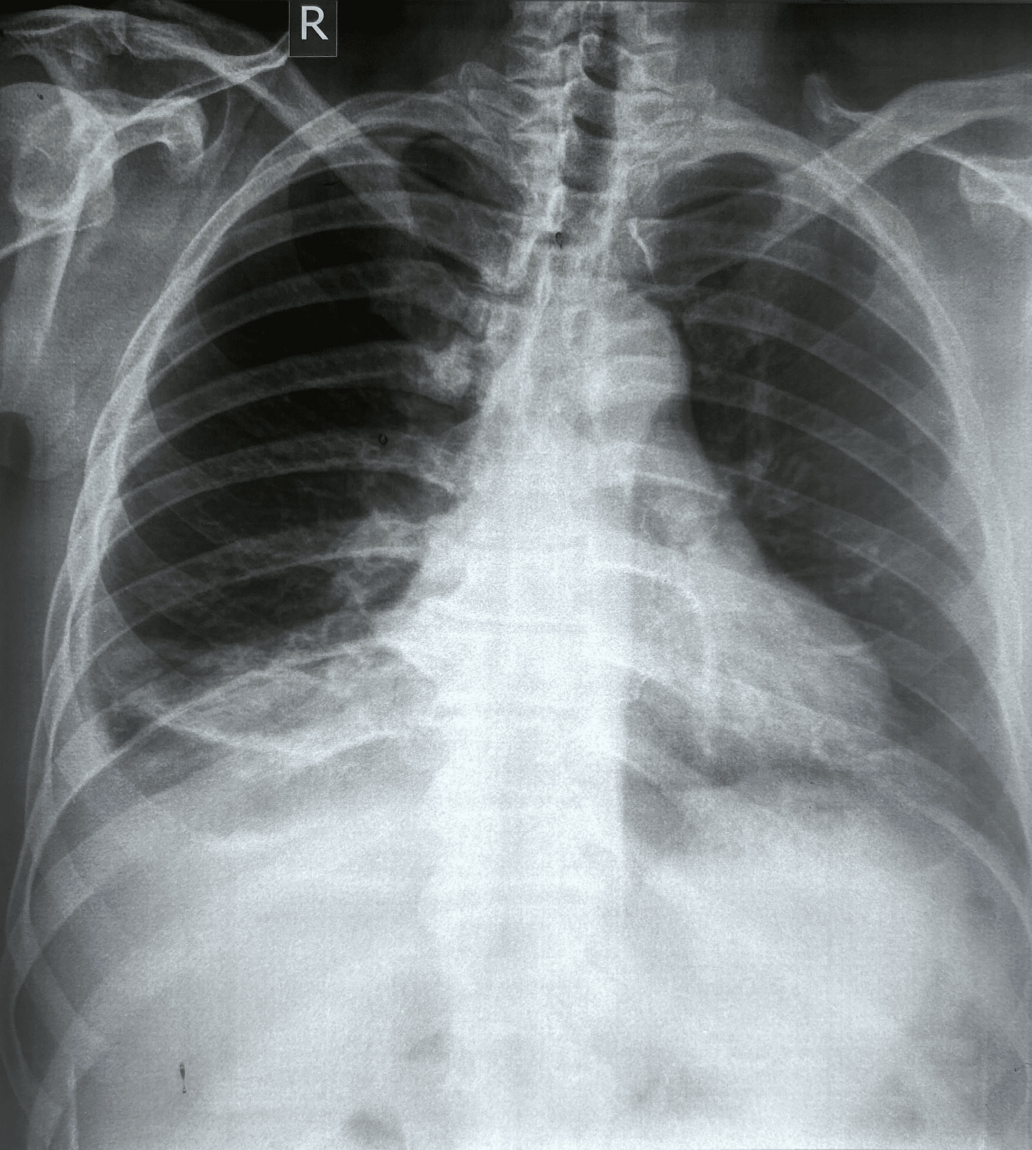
Follow-up Chest X-ray shows a marked interval improvement
